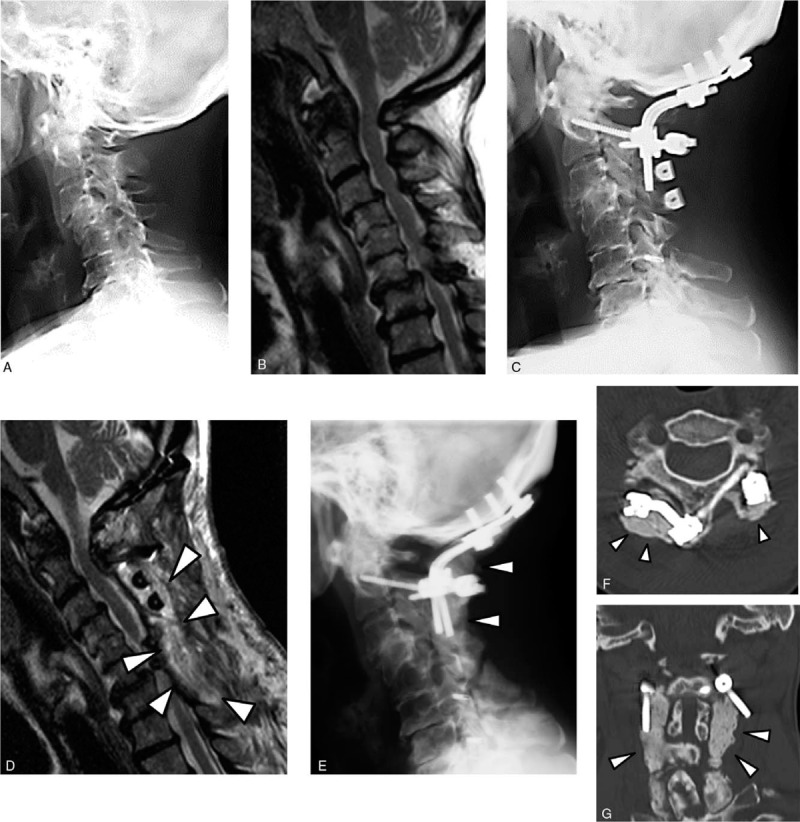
Case 3: (A) Preoperative lateral view radiograph. (B) Preoperative mid-sagittal T2-weighted MRI. (C) Postoperative lateral view radiograph. (D) Mid-sagittal T2-weighted MRI taken after the occurrence of deep SSI, revealing abscess formation in the subfascial space (*arrowhead*s). (E) Lateral view radiograph recorded following the procedure for the treatment of deep SSI. ALBC covering metal implants is shown (*arrowhead*s). (F) Axial CT image and (G) coronal CT image following the procedure for the treatment of deep SSI. The images show that ALBC embedded metal implants occupy the dead space in the operated wound (*arrowhead*s). ALBC = antibiotic-loaded bone cement, CT = computed tomography, MRI = magnetic resonance imaging, SSI = surgical site infection.
